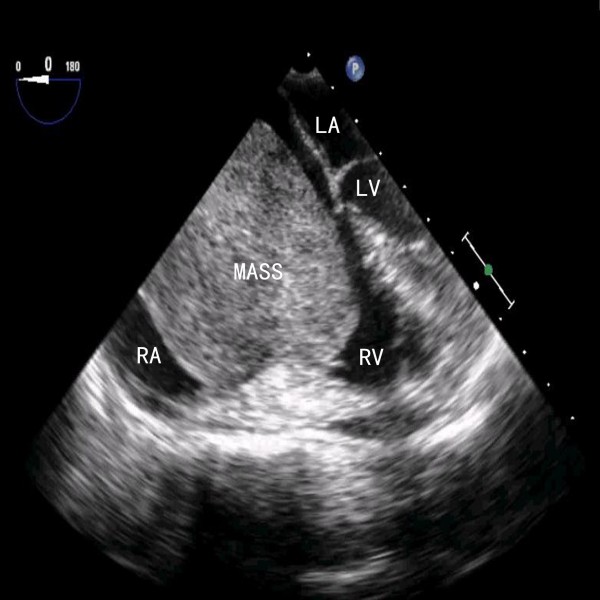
Transesophageal echocardiography demonstrated an enlarged cavity of the right atrium with the mass prolapsing through the tricuspid orifice into the right ventricle during diastole. RA, right atrium; RV, right ventricle; LA, left atrium; LV, left ventricle.
